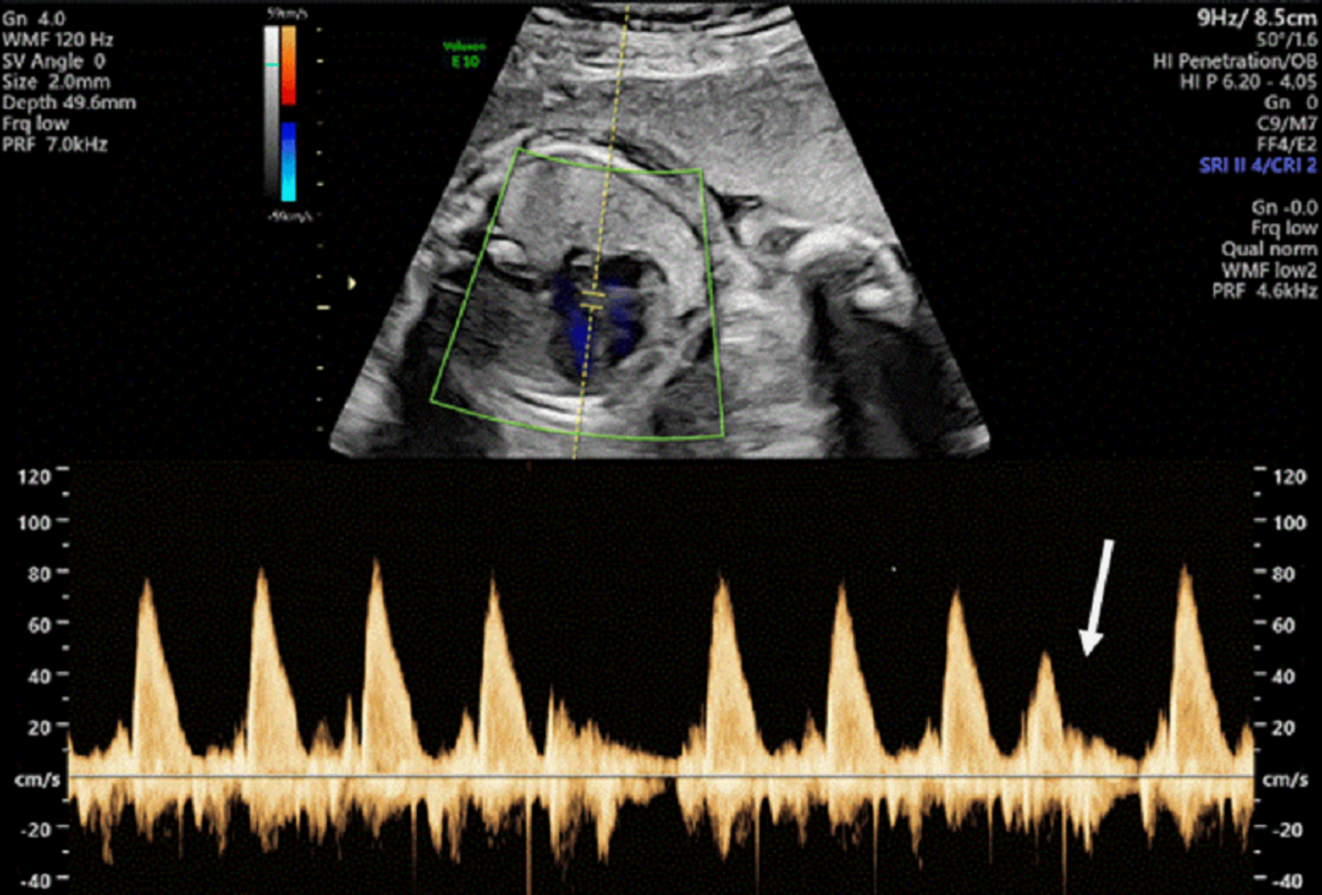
Pulsed-wave Doppler of the fetal left ventricular outflow tract showing a probable premature atrial contraction The premature beat (white arrow) is most consistent with a premature atrial contraction, suggested by the absence of a compensatory pause. However, this observation is not definitive, as the rhythm was intermittent and could not be captured on M-mode, which would have provided definitive confirmation.
